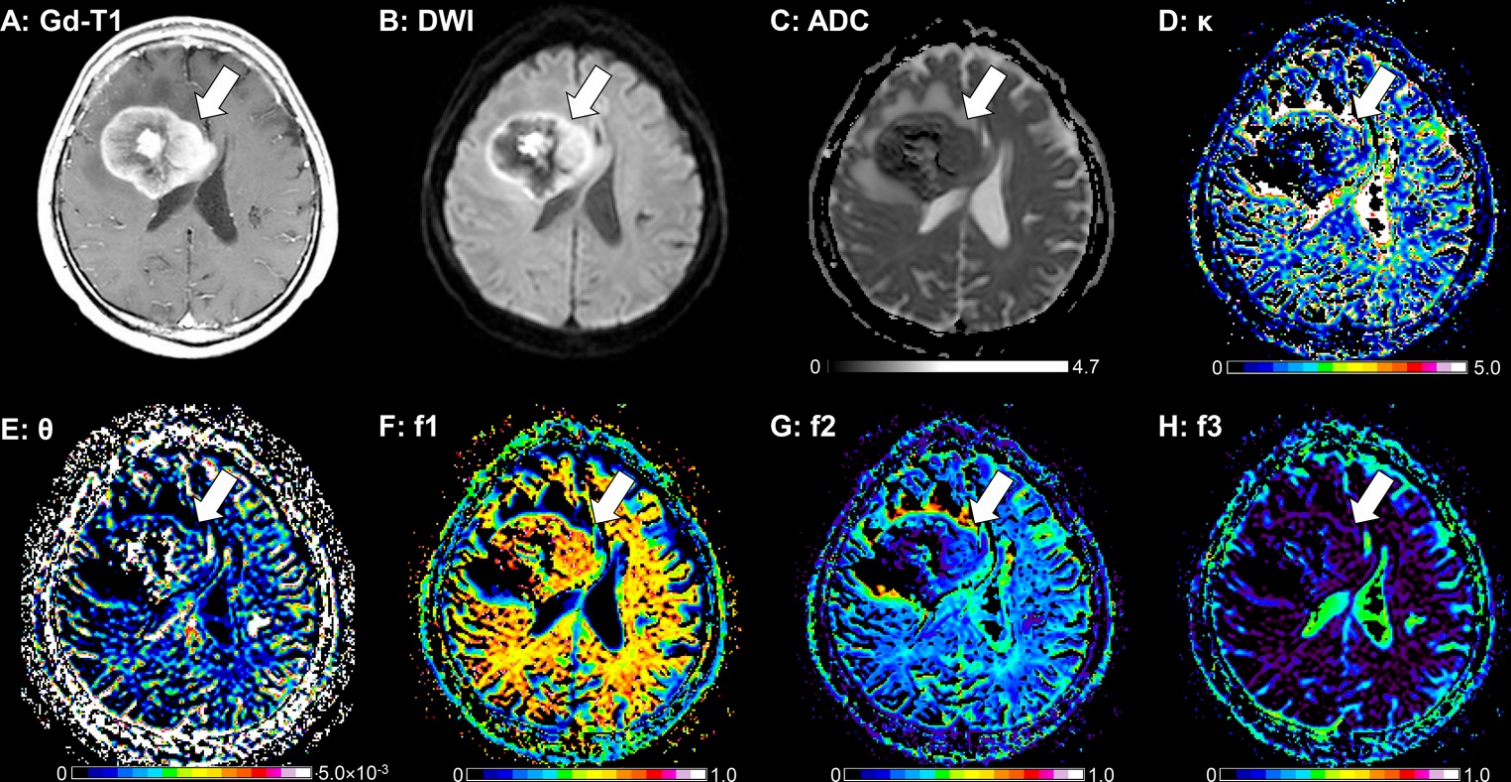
A 62-year-old-male with a PCNSL. A: The post-contrast T1-weighted image shows a ring-like
